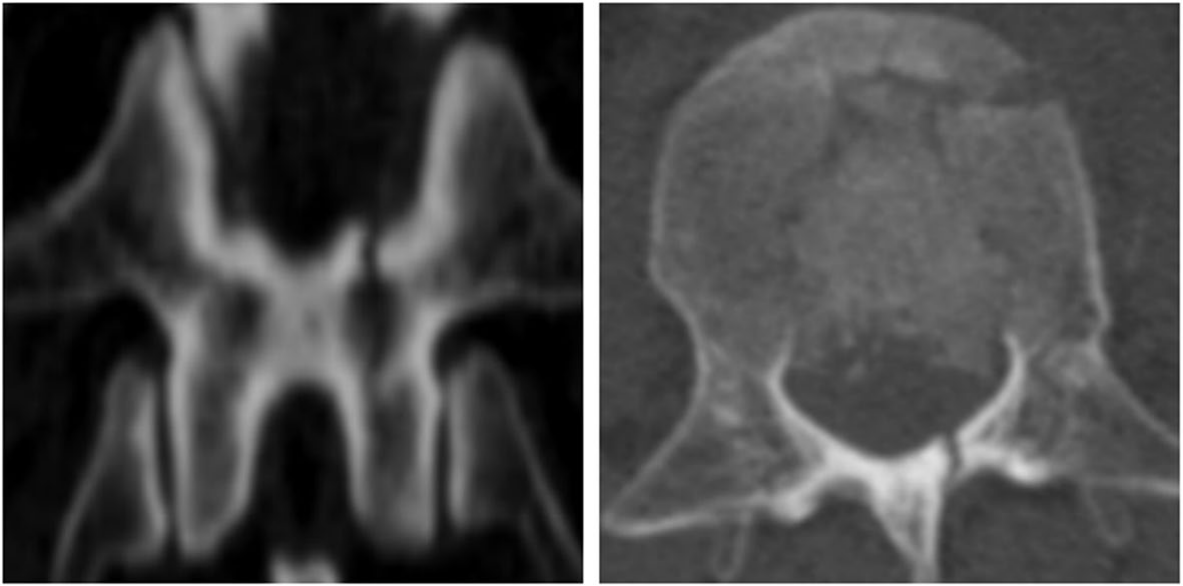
Partial and full-length laminar fractures. Reconstructed coronal computed tomographic scan revealing full-length laminar fracture. The axial plane shows complete or incomplete laminar fracture
